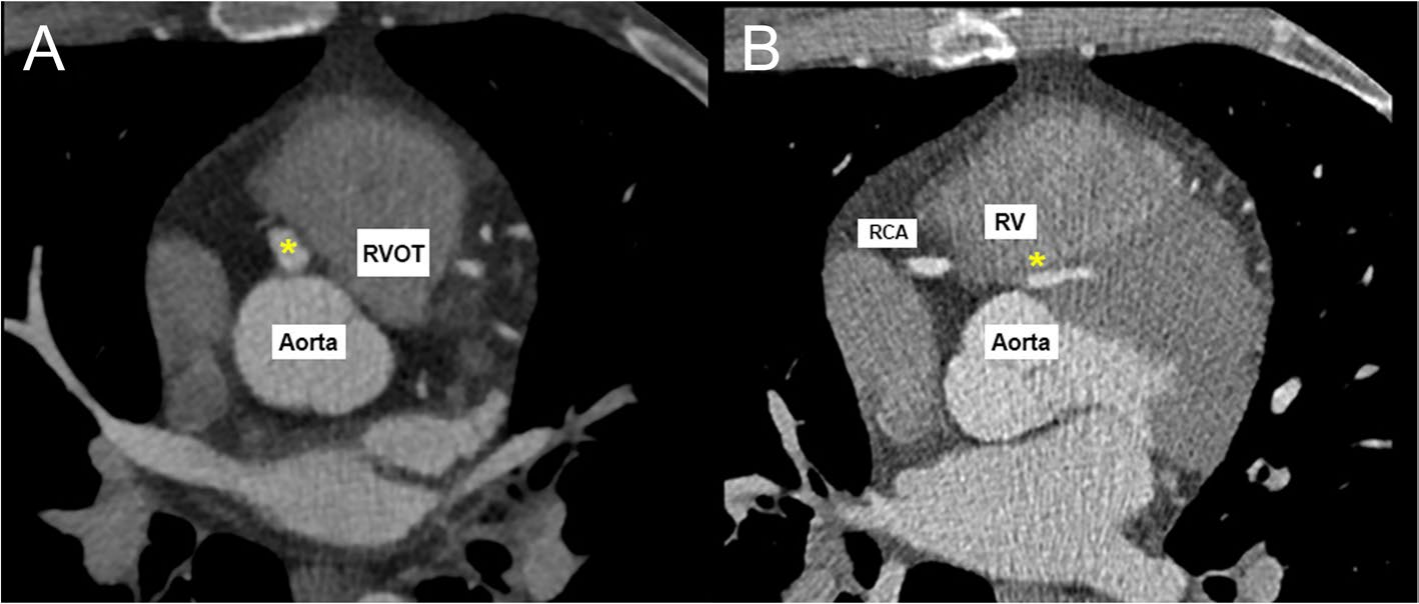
Computed Tomography Axial View. a CT Axial view showing common origin of RCA and LMCA (*). b CT Axial view showing subpulmonic and ‘malignant’ course of LMCA (*)
